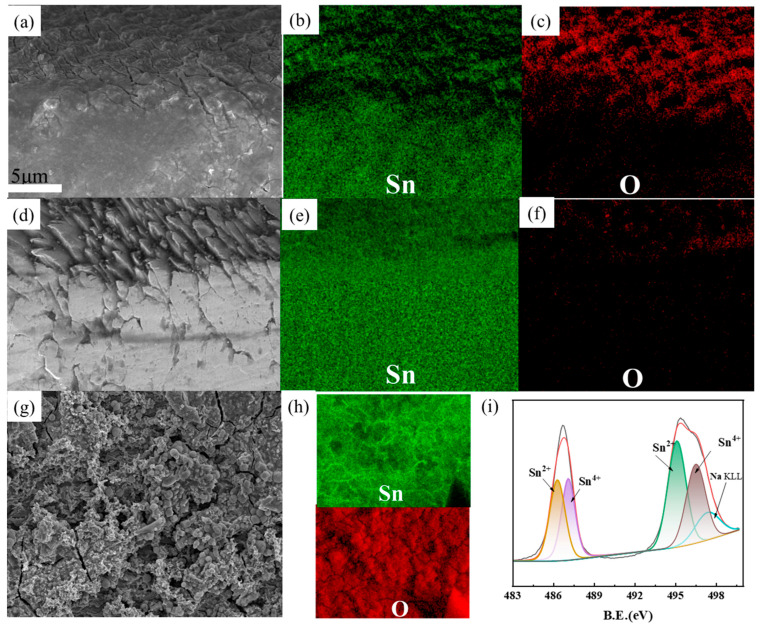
SEM images of the tin sheet passivation interface at conventional treatment (a); distribution of tin elements at the passivation interface under conventional conditions (b); distribution of oxygen elements at the passivation interface under conventional conditions (c); SEM images of the tin sheet passivation interface at ultrasonic treatment (d); distribution of tin elements at the passivation interface under ultrasonic conditions (e); distribution of oxygen elements at the passivation interface under ultrasonic conditions (f); SEM images of the tin sheet surface at conventional treatment (g); distribution of tin and oxygen elements at the passivation interface under ultrasonic conditions (h); results of the XPS analysis of the tin sheet surface at conventional treatment (i).
